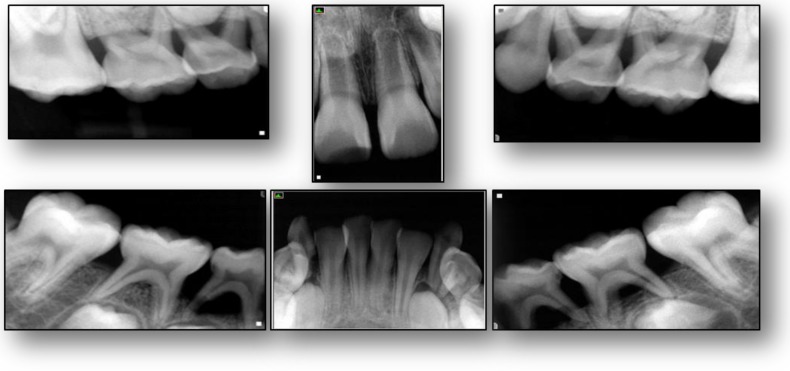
Radiographic presentation of an eight-year-old with a history of GSD type Ib. Radiographic examination reveals severe horizontal bone loss involving the remaining primary dentition.
